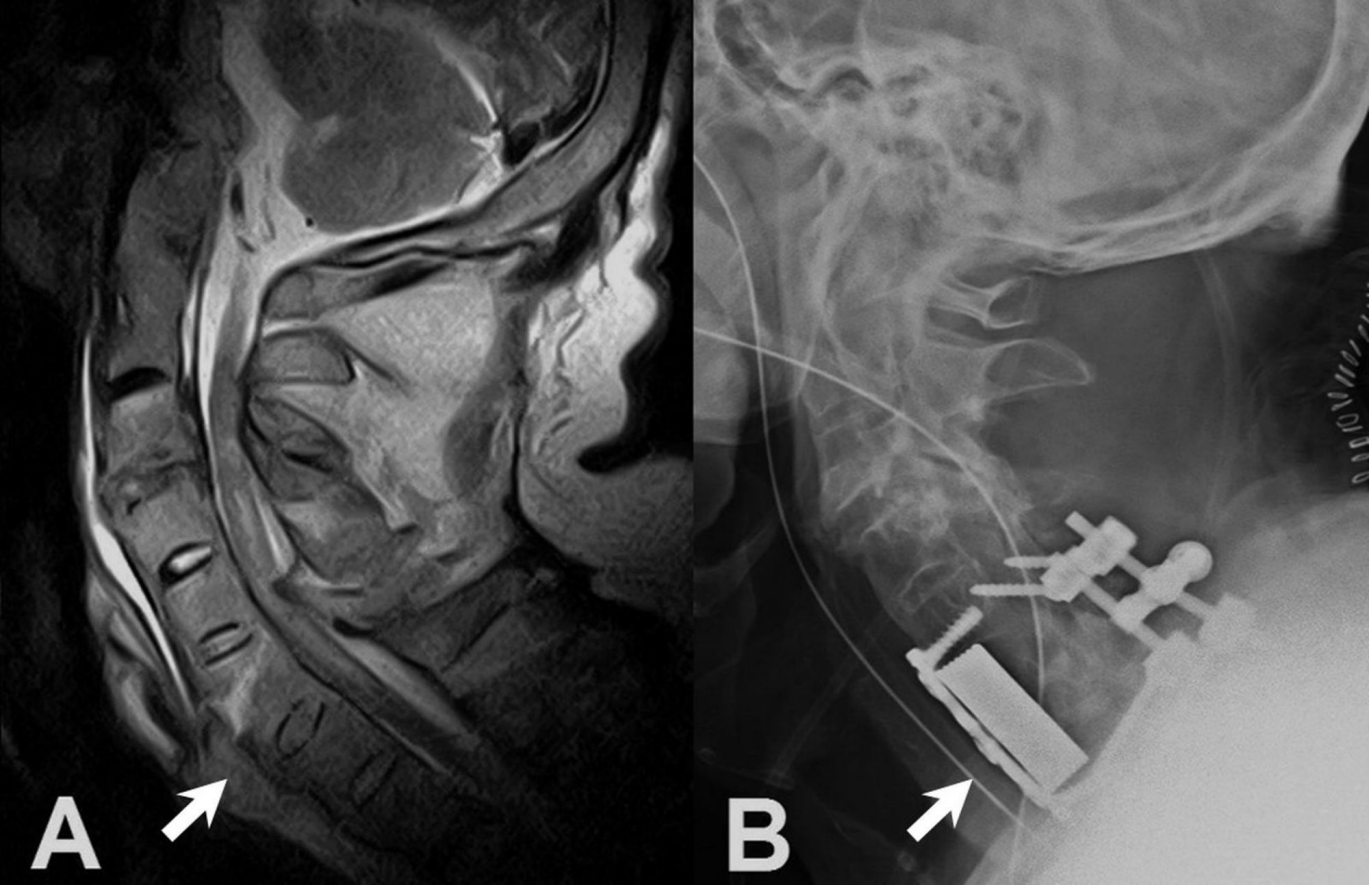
Lateral radiograph: preoperative and postoperative A: Preoperative sagittal T2-weighted cervical magnetic resonance image demonstrated remarkable spinal cord compression with increased intramedullary signal intensity, caused by a C6 burst fracture (arrow), in the subaxial cervical spine. B: Postoperative lateral radiograph demonstrated the instrumented anterior and posterior fusion, C5-6-7.
